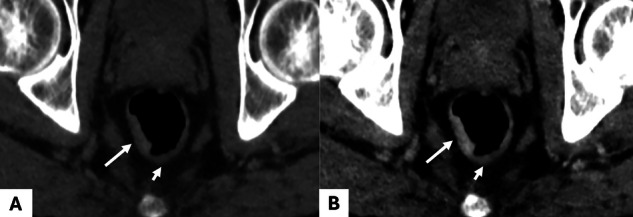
57-year-old man with T2 low rectal cancer. DS-DECT (Siemens Force) in axial section at VP. A 70 keV VMI image shows minimal attenuation difference between the tumor (long arrow) and the adjacent normal rectal wall (short arrow). B 40 keV VMI image shows increased contrast between the tumor (long arrow) and the adjacent normal rectal wall, increasing contrast-noise-ratio between the tumor and the adjacent rectal wall. DS-DECT, dual-source dual-energy computed tomography; VMI, virtual monoenergetic images
